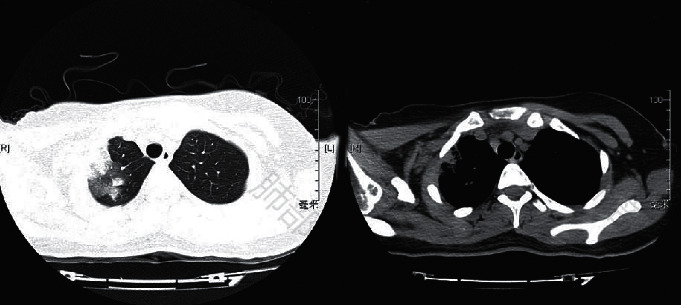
A 6-year-old female child's lung CT image.
